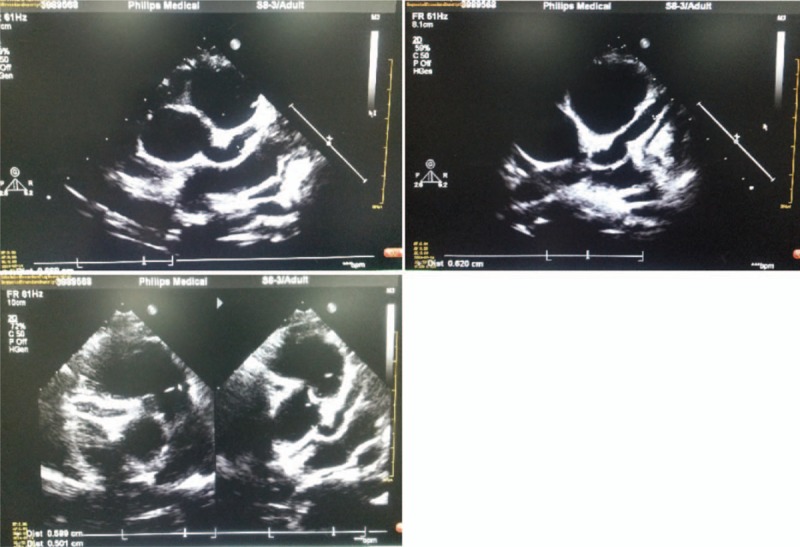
Echocardiogram pictures of case report at the diagnosis of systemic lupus erythematosus (SLE).
